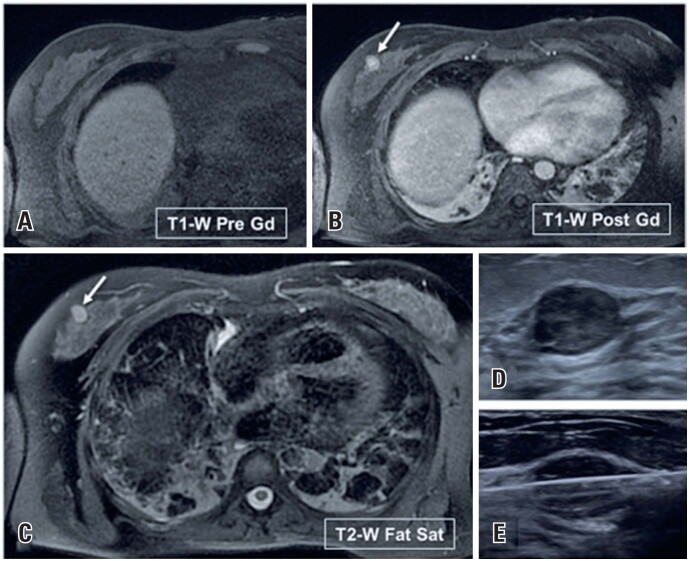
A 44-year-old woman with Crohn's disease underwent an abdominal MRI to evaluate a perianal fistula. (A to C) The MRI incidentally detected a vascularized mass in the right breast (arrows). (D and E) On ultrasound, the lesion appeared as an oval, circumscribed mass. A biopsy confirmed a Phyllodes tumor
